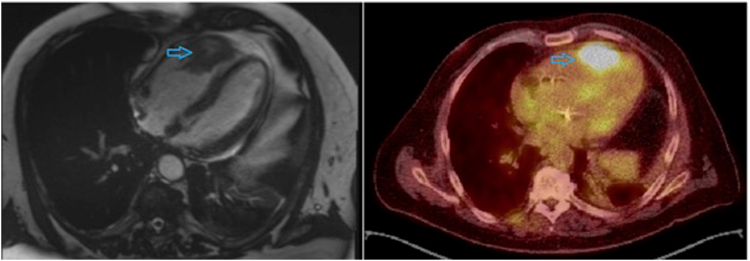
Postprocedural multimodality imaging. (Left) Cardiac magnetic resonance imaging showing a heterogeneous, solid, and partially cystic mass (blue arrow) involving the right ventricular lateral wall, extending to the apex with papillary muscle involvement. The mass exhibits postcontrast enhancement and central T2 heterogeneity suggestive of necrosis. These findings are atypical for thrombus and raise concern for metastatic disease. (Right) Positron emission tomography-computed tomography shows marked luorodeoxyglucose uptake within the corresponding right ventricular region (blue arrow), with a maximum standardized uptake value of 15.37, consistent with high metabolic activity and further supporting the diagnosis of malignancy. Additional findings included a hypermetabolic pretracheal lymph node and a posterior neck cutaneous focus, indicating further sites of concern. Multimodal imaging collectively supported the diagnosis of intracardiac metastasis.
